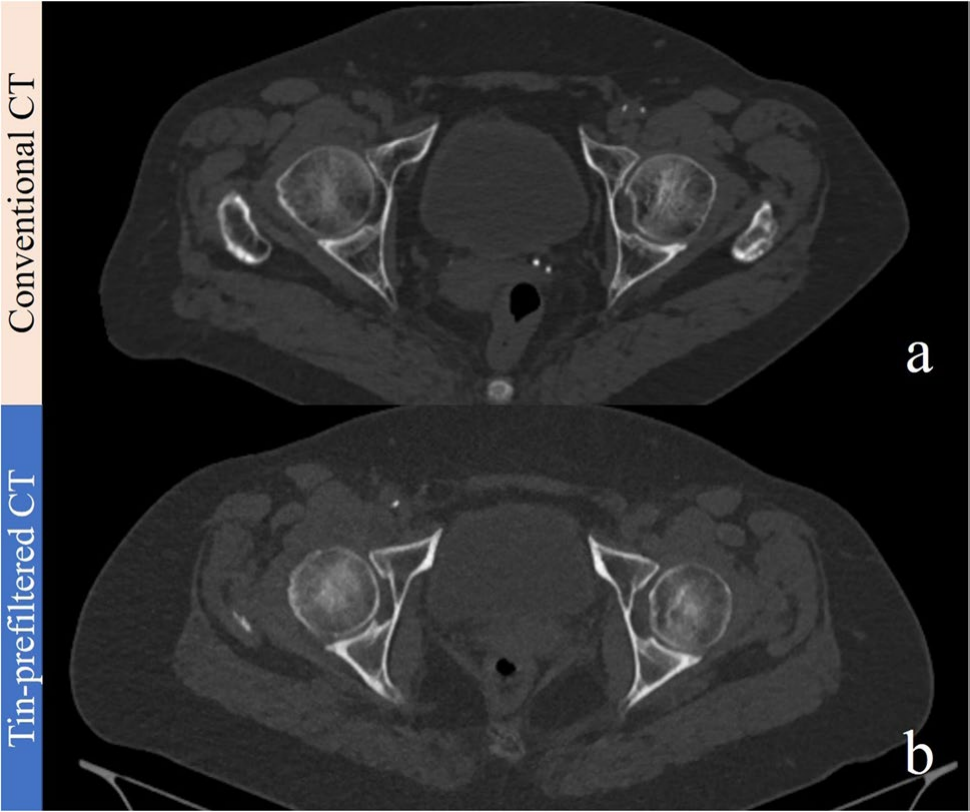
Conventional CT scan of the pelvis of a 71-year-old female (BMI 26.6 kg/m^2^) (a) compared to a tin-prefiltered CT scan of the pelvis of a 61-year-old female (BMI 26.6 kg/m.^2^) (b). CTDI_vol_ of the tin-prefiltered scan was 4.04 mGy compared to 11.3 mGy of the conventional CT scan. All images were reconstructed with the convolution kernel BR38, slice thickness of 1 mm, iterative reconstruction strength level 3, and displayed at a window/center level of 1500/450
